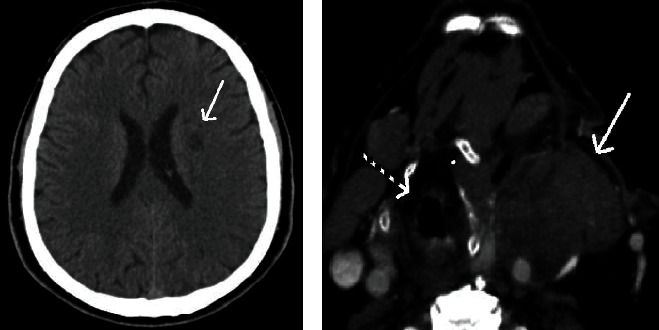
Computed tomography showing (a) a small ischemic lesion in the left white matter (arrow) and (b) a large cervical hematoma (white arrow) with deviation of the trachea (interrupted arrow).
